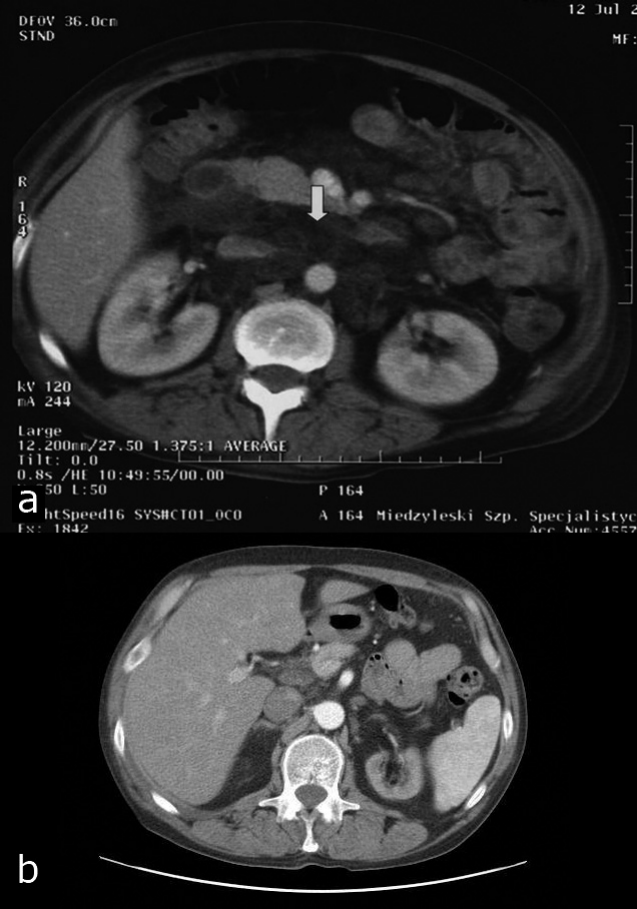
Computed tomography scans of the abdomen shows enlargement of mesenteric and retroperitoneal lymph nodes with high amount of lipids (exacerbation of Whipple disease) (A). Marked reduction of lymph nodes on one-year follow-up (B).
